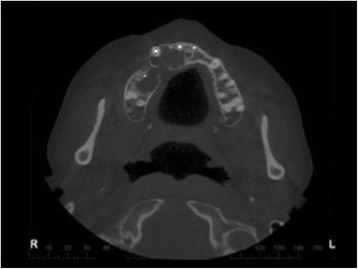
Axial image from computed tomography scan showing lesion divided by bony septum
